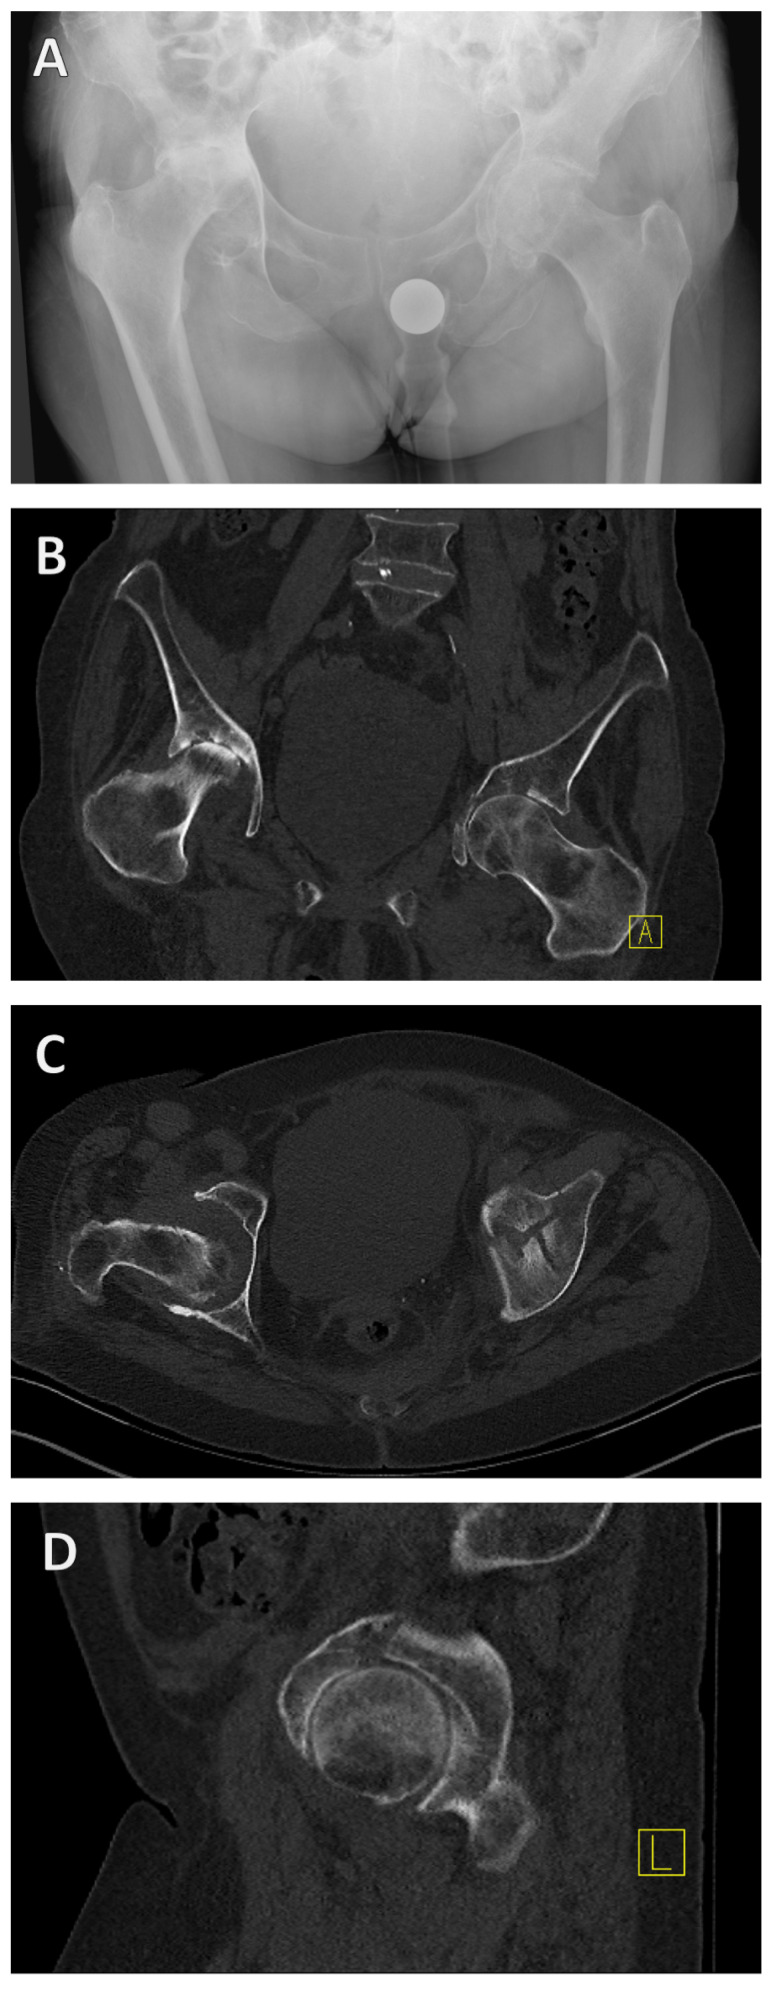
X-ray and CT diagnosis of a geriatric acetabular fracture. Initially, the left-sided acetabular fracture can be recognized in the anterior–posterior radiograph of the pelvis (A). A CT scan was added for fracture classification and preoperative planning (B–D). It revealed a T-shape fracture (B–D).
